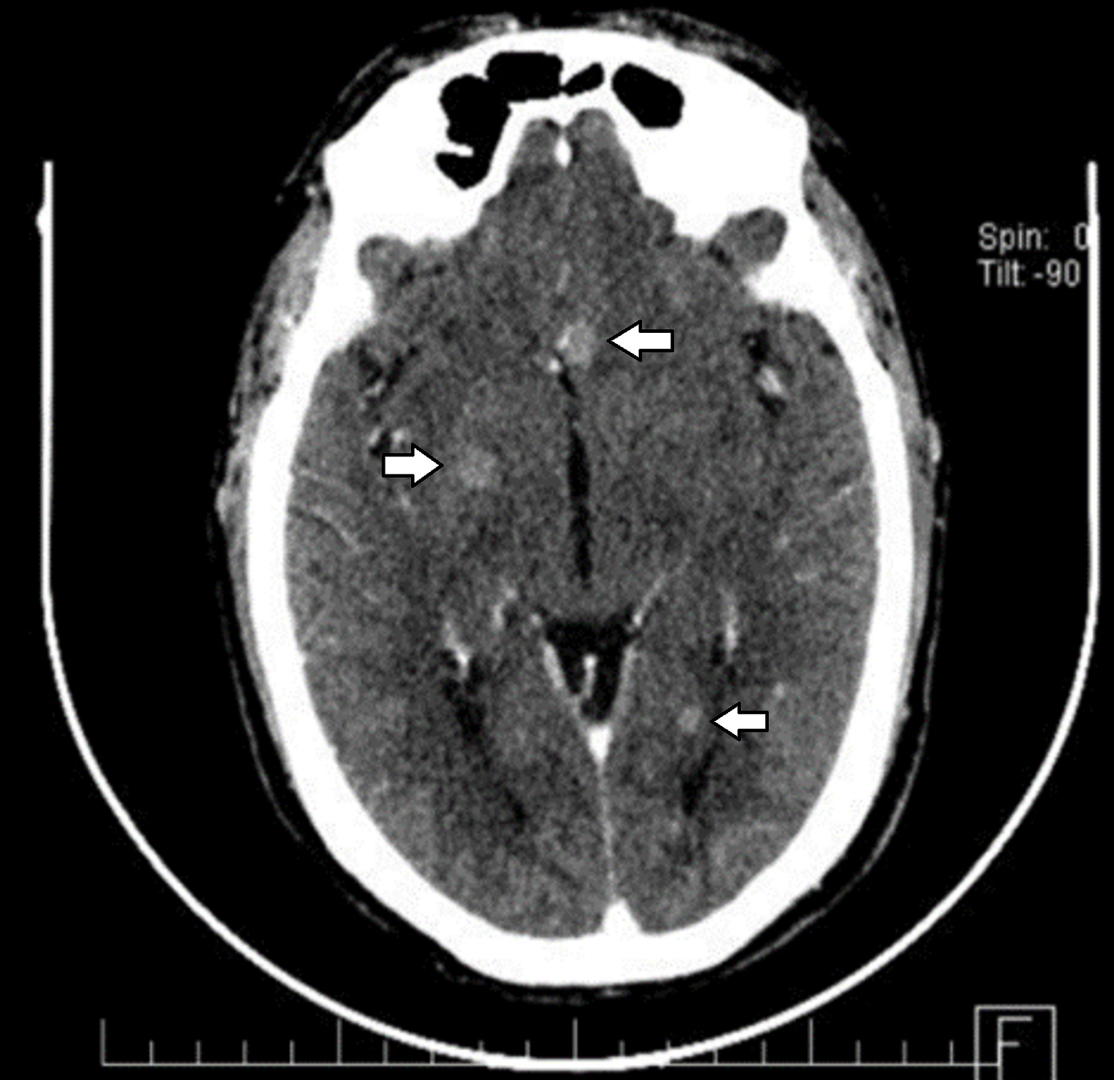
Computed tomography of the brain Three nodular brain lesions with contrast enhancement
